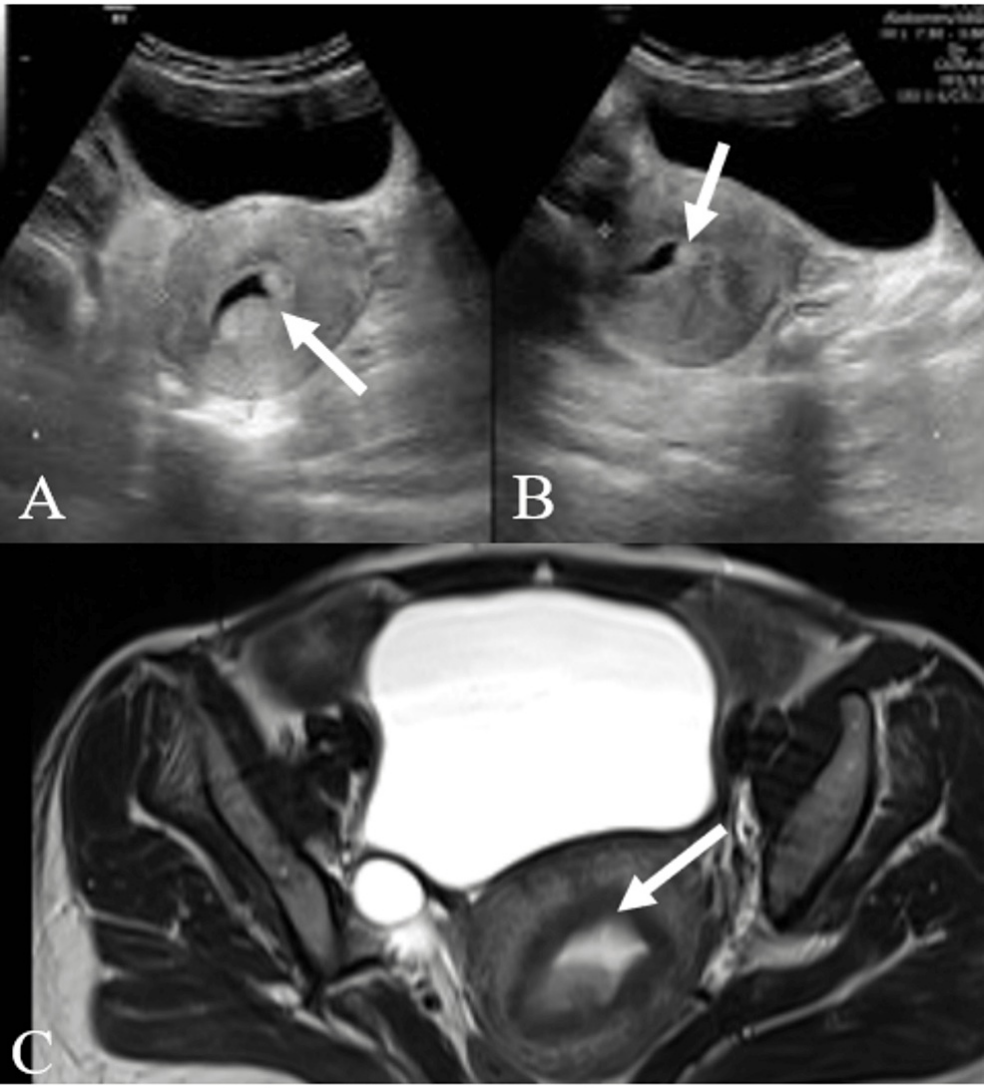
A case of endometrial polyp A and B: USG of the pelvis transverse and longitudinal sections showing two hyperechoic lesions (white arrow) in the endometrial cavity. C: MRI of the pelvis T2 weighted image showing three isointense lesions (white arrow) within the endometrial cavity. USG - ultrasonography
